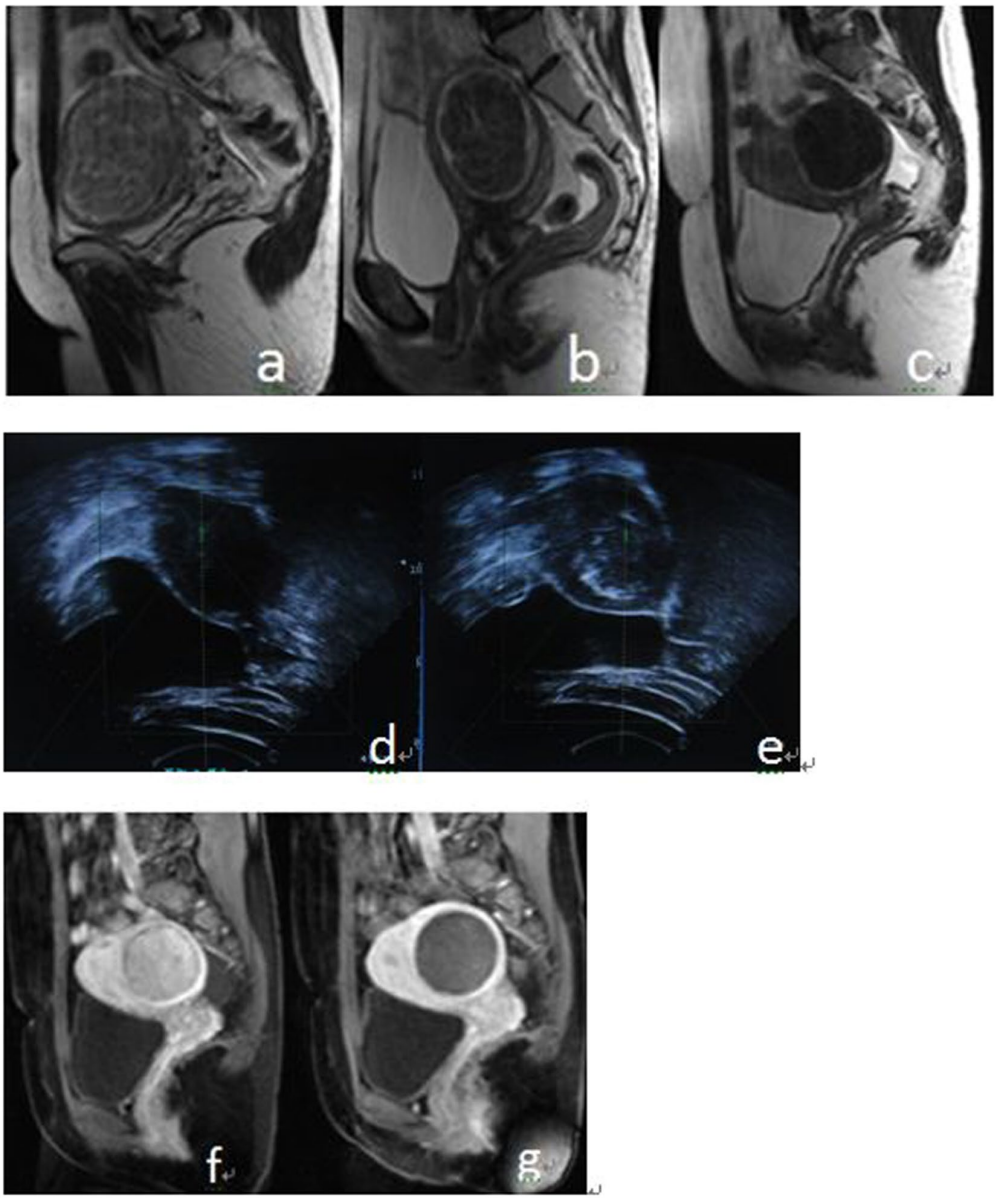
(a–c) MRI appearance of different types of uterine fibroids on T2-weighted sagittal MRI before treatment; (a) hypointense; (b) isointense; (c) hyperintense. (d–e) US images that show the ablation volume during the real-time USgHIFU; (d) ultrasound image shows a uterine fibroid with hypoecho before treatment; (e) gray scale changes was observed after treatment. (f–g) Enhanced sagittal MRI before and after treatment, the NPV is visible inside uterine fibroids; (f) before treatment, g after treatment.
